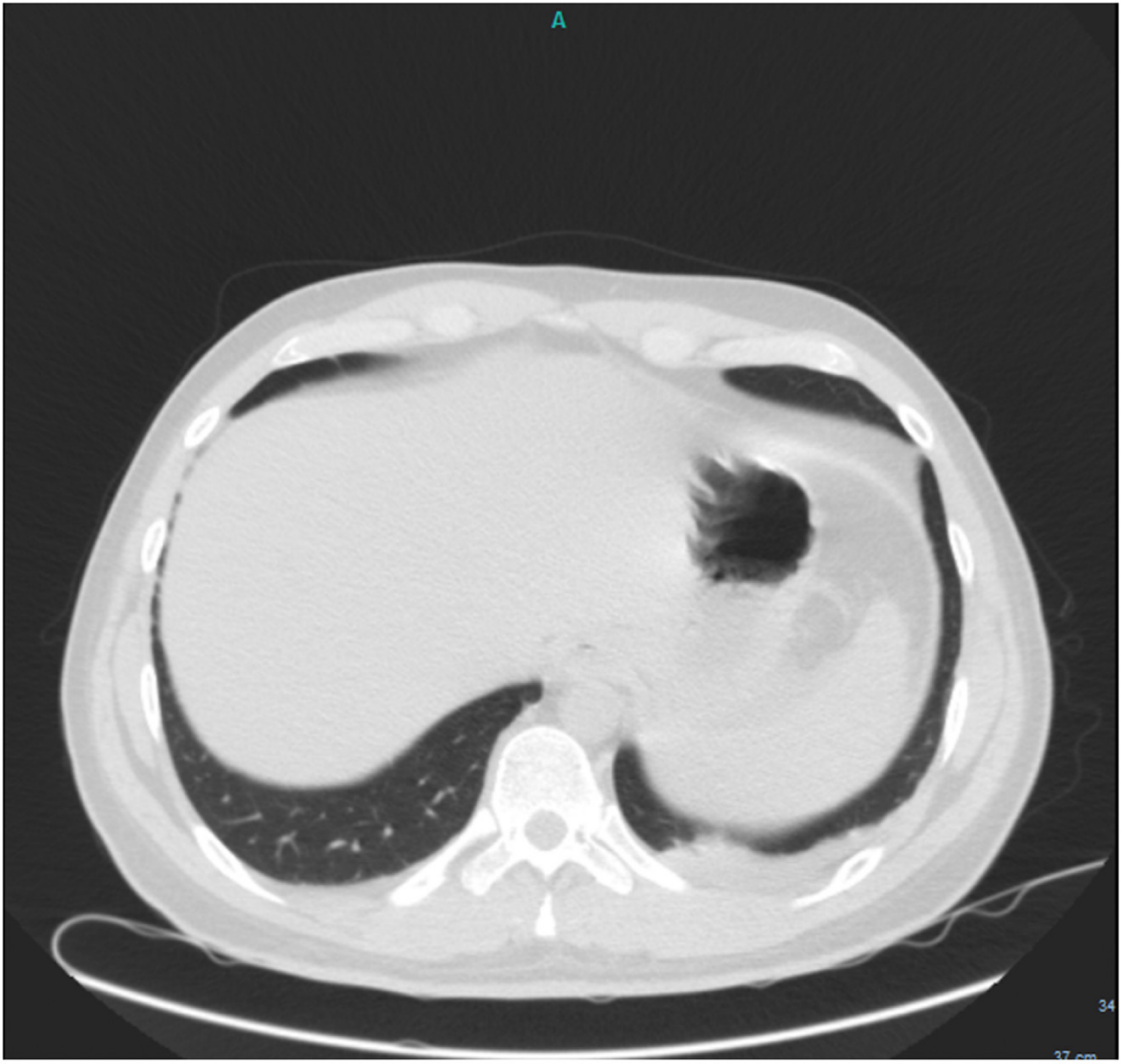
Chest computed tomography scan, pulmonary window, axial, showing left‐side pleural effusion.
